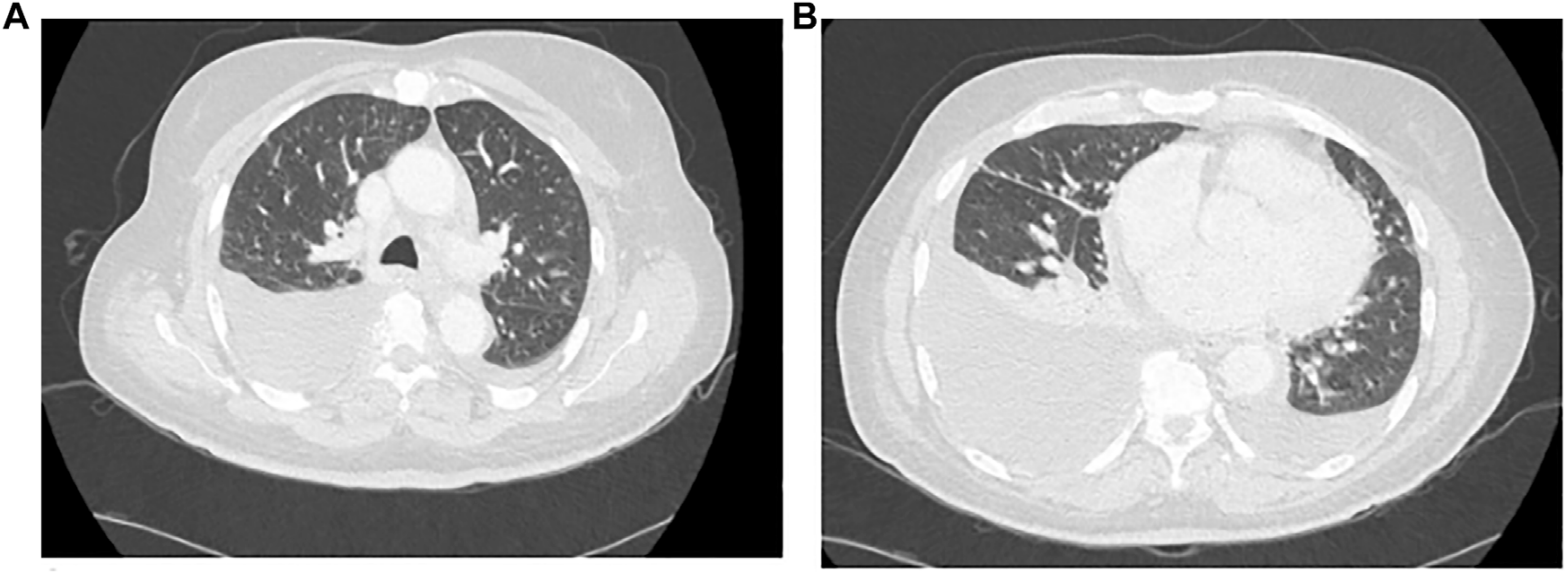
Chest CT Scan, (A) Thoracic high, (B) Thoracic low.
